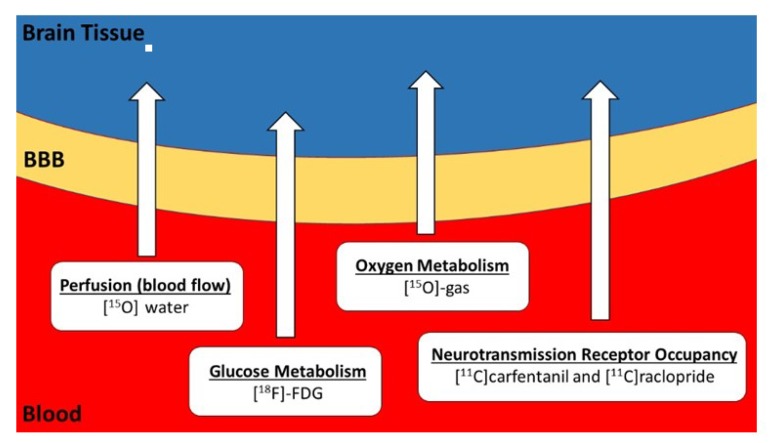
Potential PET tracers available for combination with tDCS. Glucose is the most important energy resource of the human brain and its metabolism can be measured and quantified using positron emission tomography (PET) with [^18^F]-fluorodeoxyglucose (FDG). Oxygen is necessary for the operation of the tricarboxylic acid cycle to synthesize ATP molecules from glucose, and oxygen metabolism can be measured using PET with [^15^O]-oxygen gas during inhalation. Glucose and oxygen molecules are supplied by blood flow. Brain regions with increased activity are accompanied by regional capillary dilation and increased regional cerebral blood flow (rCBF), which can be measured with [^15^O]water PET. The interaction of neurotransmitters and receptors can also be measured using PET with various [^11^C]-labeled ligands, such as [^11^C]carfentanil and [^11^C]raclopride. BBB = blood brain barrier.
